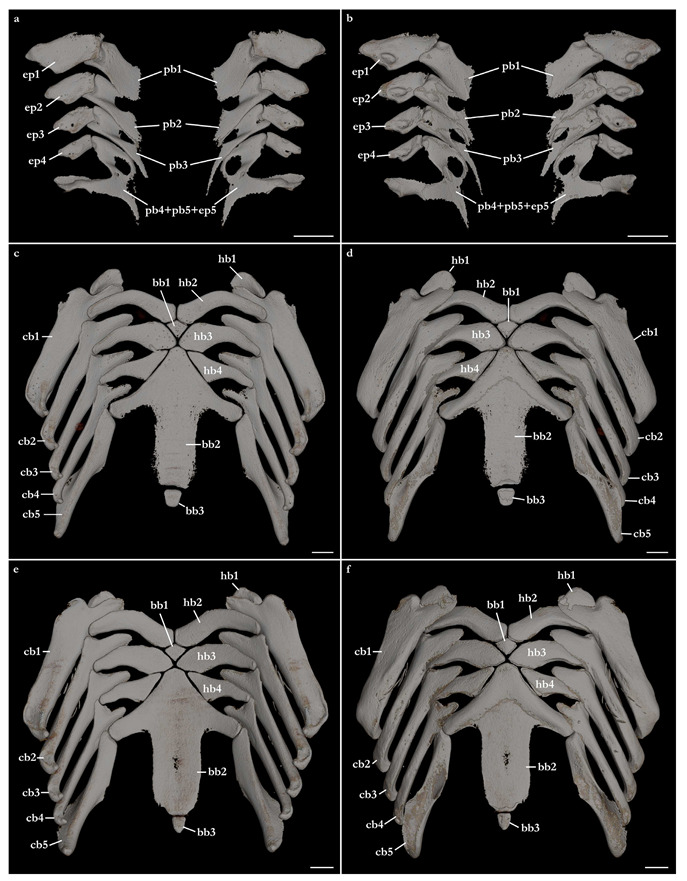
Micro-computed tomography scans of the branchial arches of (a–d) *Squatina leae* sp. nov., paratype, ZMH 26098, juvenile male, 249.6 mm TL and (e,f) *S. africana*, ZMH 123064, juvenile female, 309 mm TL. (a,b) Epi- and pharyngobranchials in (a) dorsal and (b) ventral views, (c–f) basi-, cerato-, and hypobranchials in (c,e) dorsal and (d,f) ventral views. Abbreviations: bb1–bb3, basibranchials 1–3; cb1–5, ceratobranchials 1–5; ep1–5, epibranchials 1–5; hb1–4, hypobranchials 1–4; pb1–5, pharyngobranchials 1–5. Anterior to top; scale bars: 5 mm.
